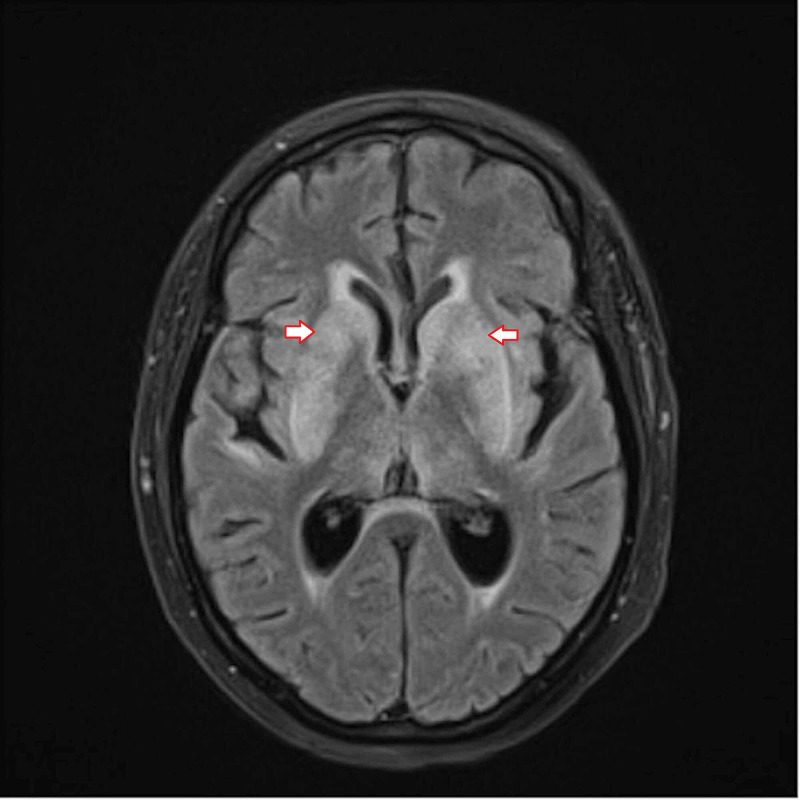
Brain MRI on initial presentation Grossly symmetric high T2 fluid-attenuated inversion recovery (FLAIR) signal abnormality involving bilateral basal ganglia (with greater involvement of caudate nuclei and putamen than globus pallidus internus), thalami, portion of corpus callosum, portion of medial temporal lobes, and perisylvian/insular cortex regions.
